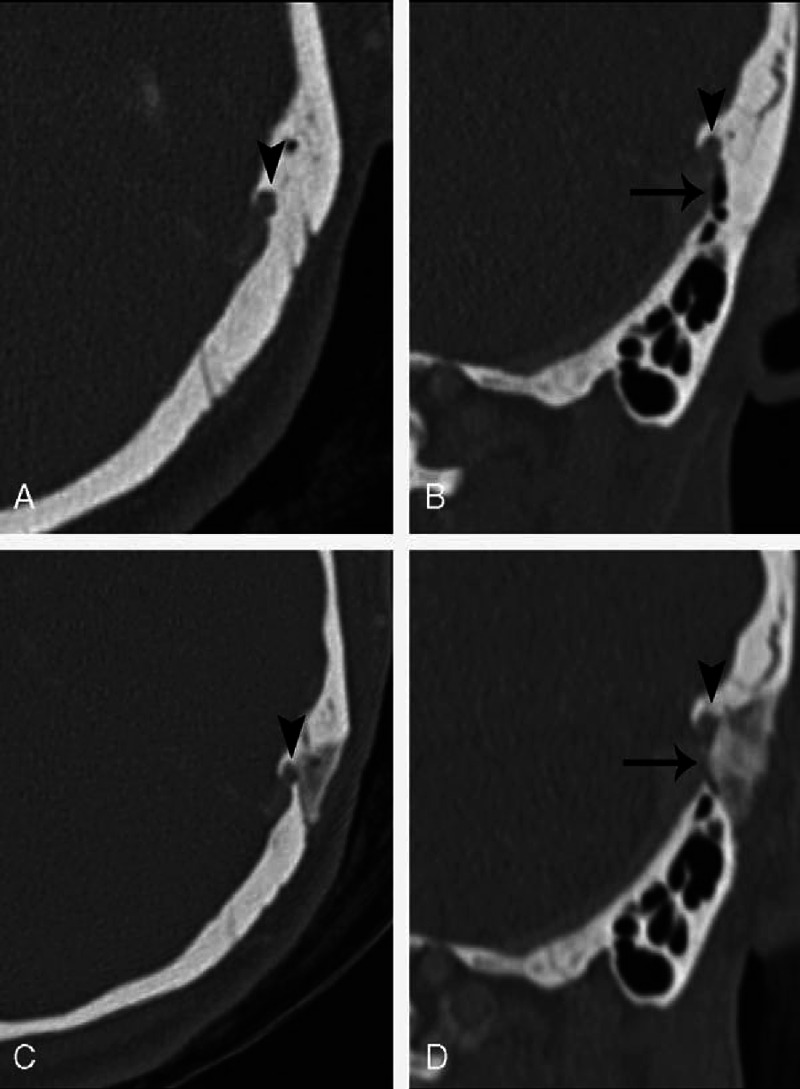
Preoperative (A and B) and postoperative (C and D) CTA images of a patient (Patient 5) experiencing the complete resolution of PT after surgery. With regard to approximate layers, (A) corresponds to (C), and (B) corresponds to (D). After surgery, the diverticulum (arrowheads) remains, but the dehiscent wall (arrows) is well reconstructed.
